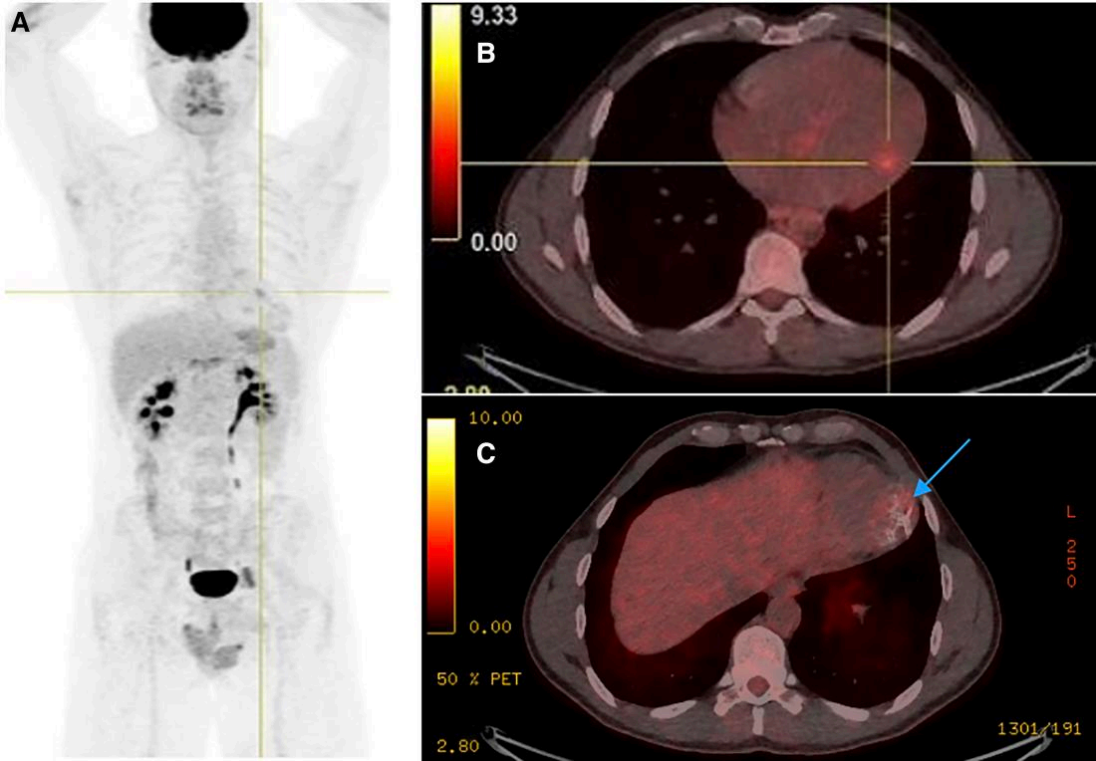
(*A*) Fluorodeoxyglucose F-18 positron emission tomography (PET), coronal view showing physiological distribution of fluorodeoxyglucose F-18. (*B*) Positron emission tomography-computed tomography axial view demonstrating moderate uptake localizing to a normal papillary muscle (presumed physiological). (*C*) No evidence of pathological uptake throughout the myocardial mass (blue arrow).
